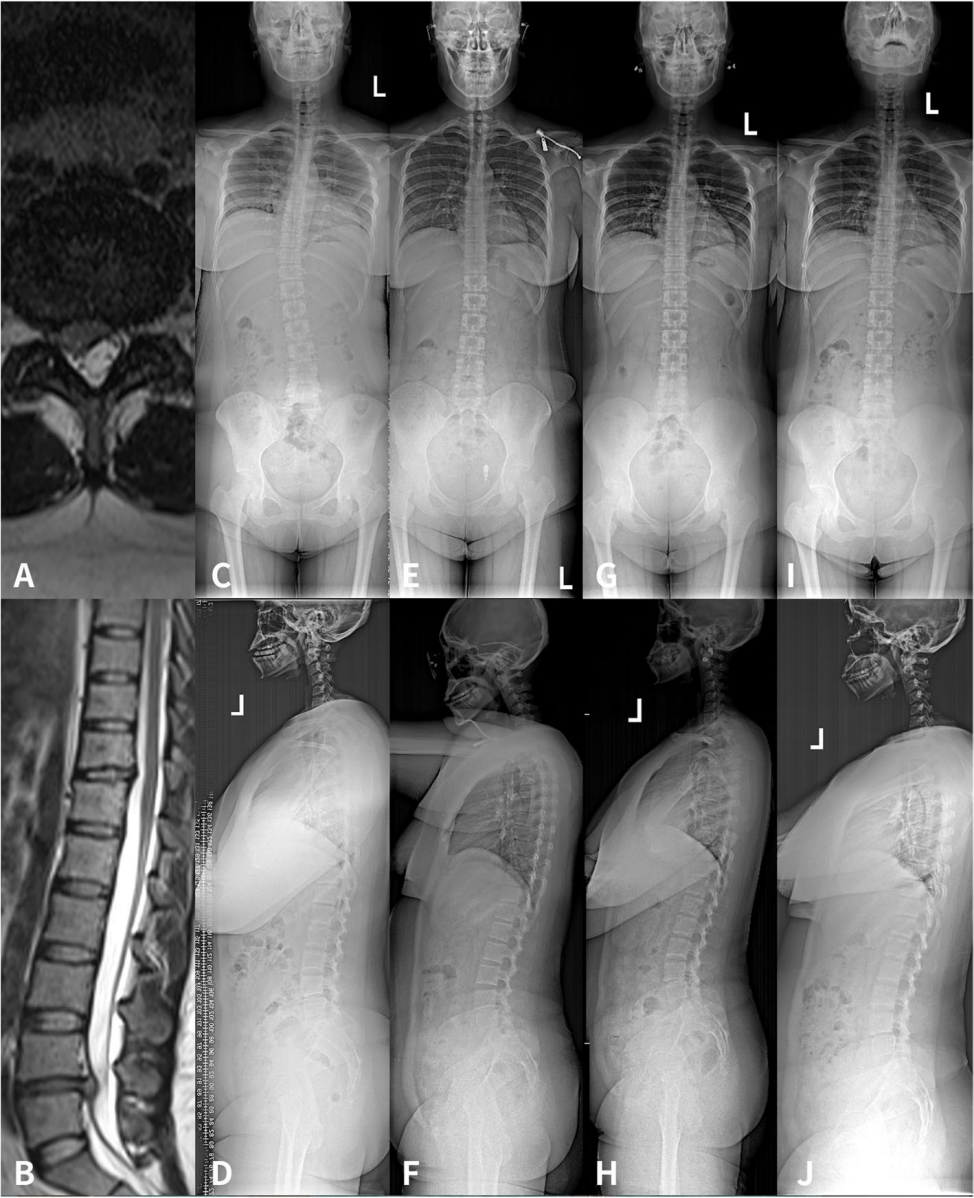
A 36-year-old female was admitted with “recurrent low back pain and right lower limb pain for more than half a year”. She was diagnosed as lumbar disc herniation at L4/5 and was treated with PELD in our hospital. A-D were preoperative lumbar magnetic resonance and anterior and lateral X-ray of the whole spine, Cobb angle: 17.5 °; AVT:22.6 mm, CBD:5.5 mm, SVA:11.4 mm, TK:11.8 °, LL:44.6 °, PI:39.7 °; E-F: postoperative anterior and lateral X-ray of the whole spine, Cobb angle: 5.7 °, AVT:16.7 mm, CBD:5.3 mm, SVA:37.5 mm, TK:13.2 °, LL:48.1 °. G-H were positive and lateral X-ray of the whole spine at 1 month follow-up, Cobb angle was 2.3°, AVT:7.8 mm, CBD:4.9 mm, SVA:35.0 mm, TK:14.1 °, LL:50.7 °, I-J Cobb angle 2.1°, AVT:5.7 mm, CBD:4.1 mm, SVA:29.1 mm, TK:15.9 °, LL:55.2°
